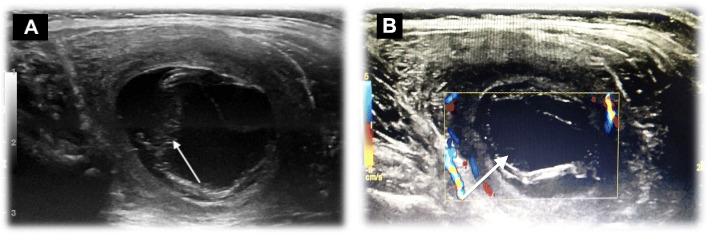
Ultrasound exam of the right Paraspinal region. a: A well-defined thick encapsulated cystic lesion is seen in the right paraspinal muscle measuring about 2.4 × 1.7 cm with water Lilly sign (arrow) consistent with hydatid cyst, b Doppler ultrasound of the same lesion showing hyperemia of the capsule and the daughter cysts (arrow)
